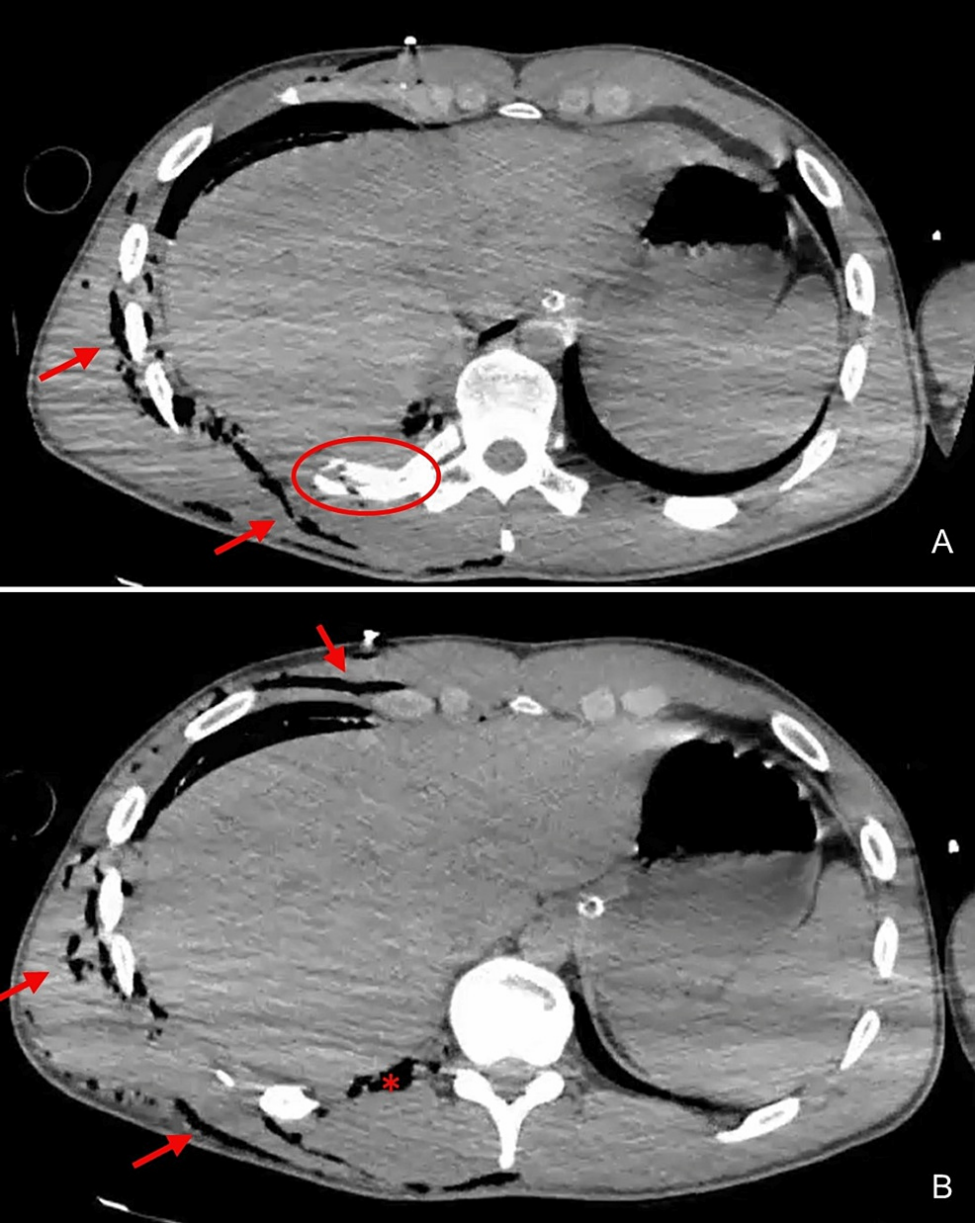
Axial CT acquisitions showing a posterior misaligned fracture of the right 10th rib (circle), extensive subcutaneous emphysema involving the right thoracic wall and extending to the neck and ipsilateral abdominal wall (arrows), and area of detachment of the diaphragm from the posterior thoracic wall (*) next to the fractures rib.
